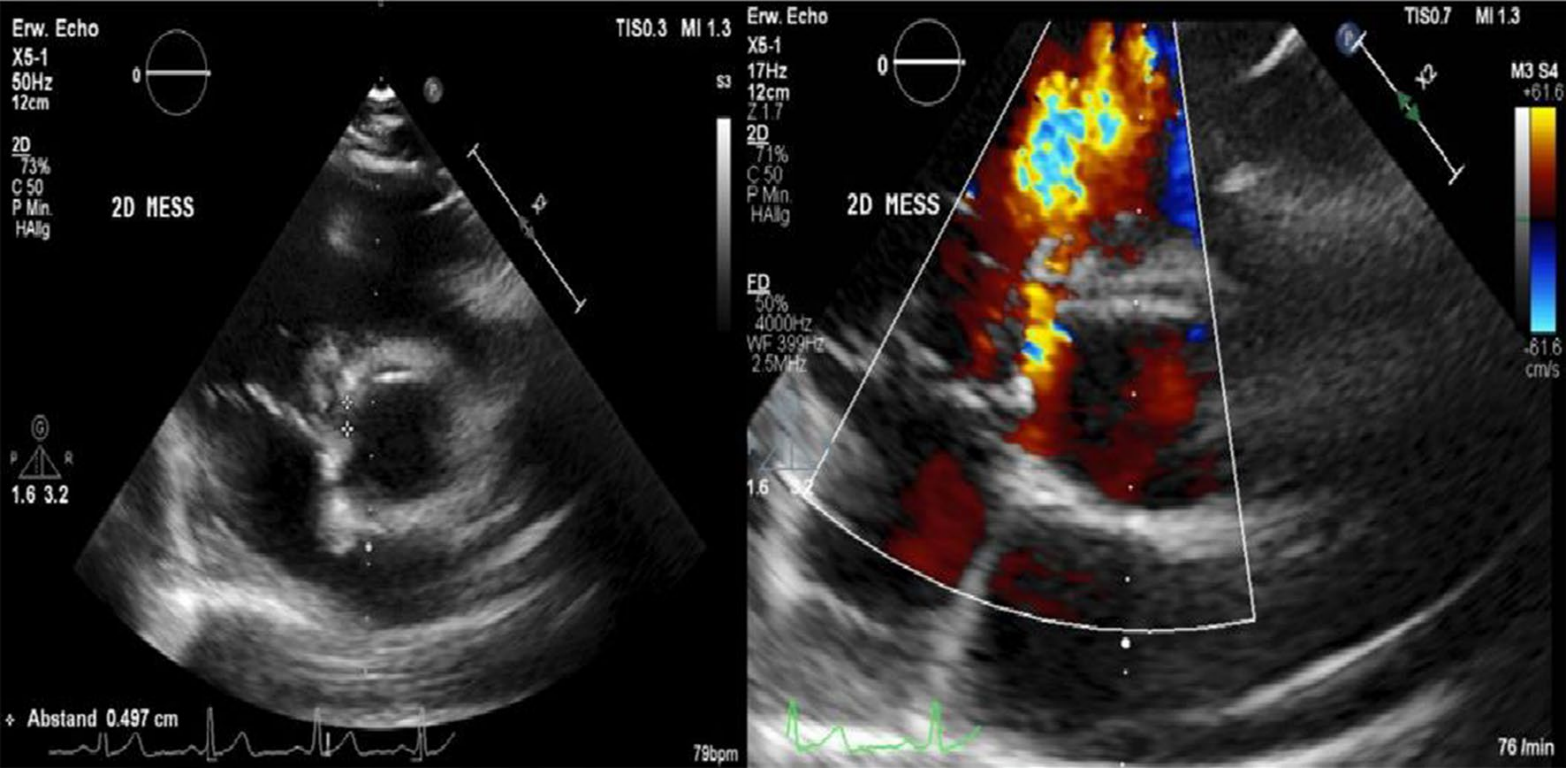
Transoesophageal echocardiography at the 4-week follow-up showed a 5 mm VSD and a left-to-right shunt
